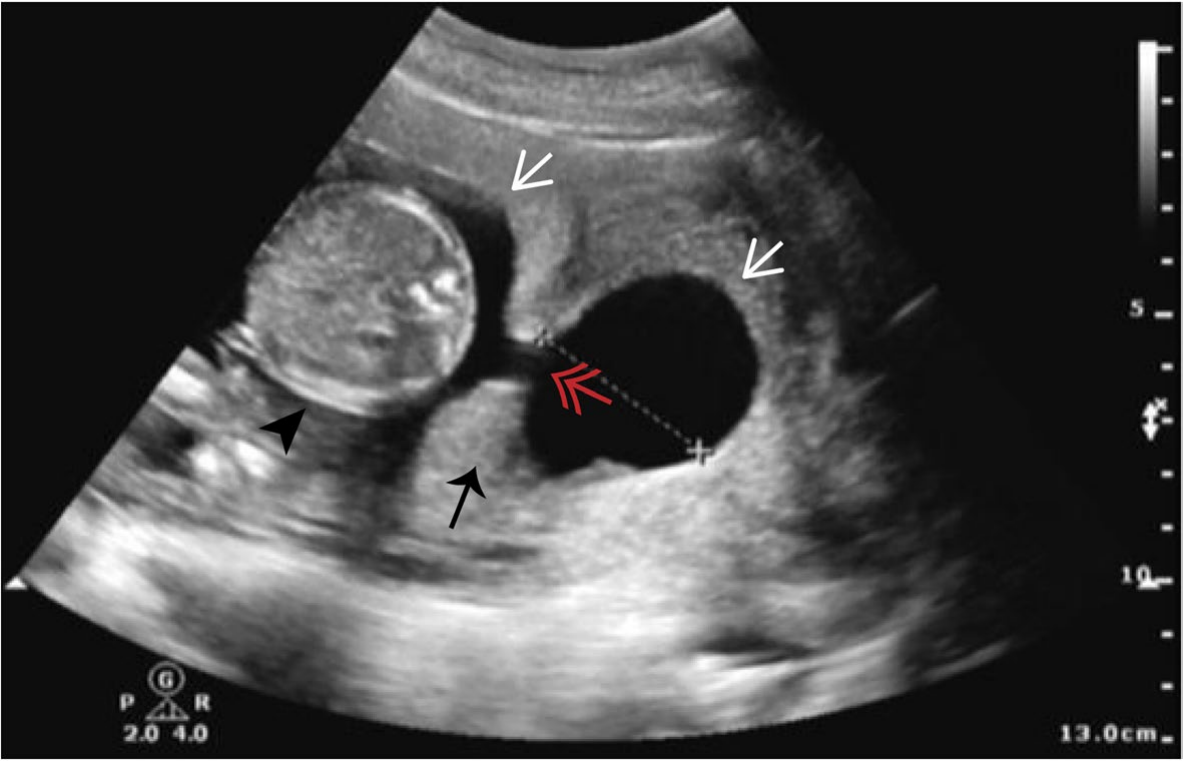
Ultrasonography for diagnosing fetal malformations. The two hemicavities (white arrows) are connected by a 10 mm hole in diameter (red double arrow) in the uterine septum (black arrow). Pregnancy occurs in the right blind hemicavity (black arrow head)
